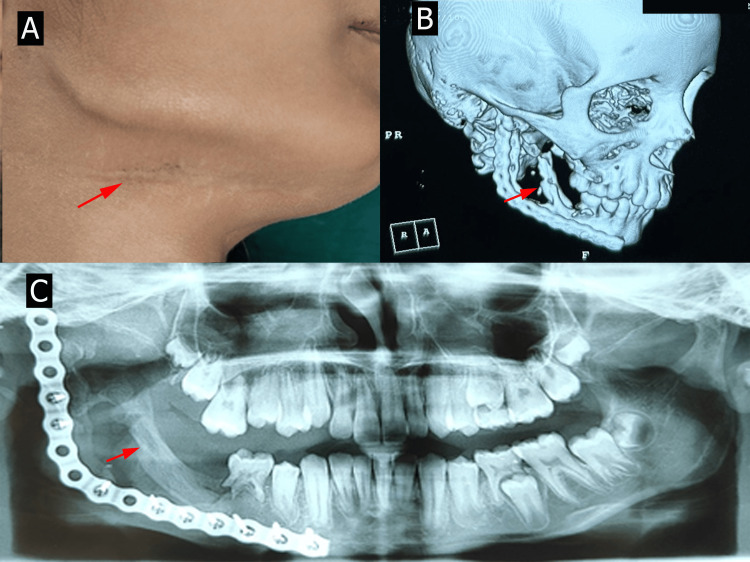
Postoperative follow-up showing (A) healed sutures and (B) satisfactory graft integration and cortical bone remodeling after six months on CBCT and (C) after one year on OPG CBCT: cone-beam computed tomography; OPG: orthopantomogram All images are original and obtained by the authors
